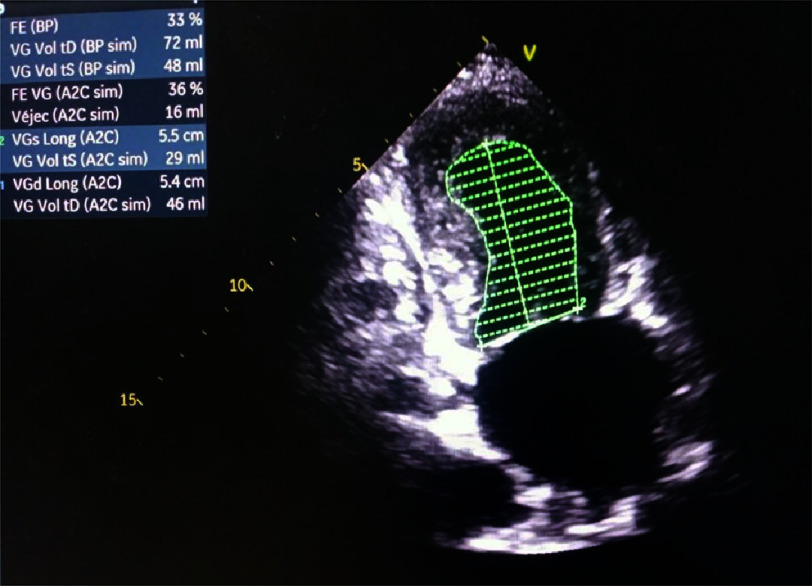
Apical two-chamber echocardiography showing left ventricular fraction at admission.
